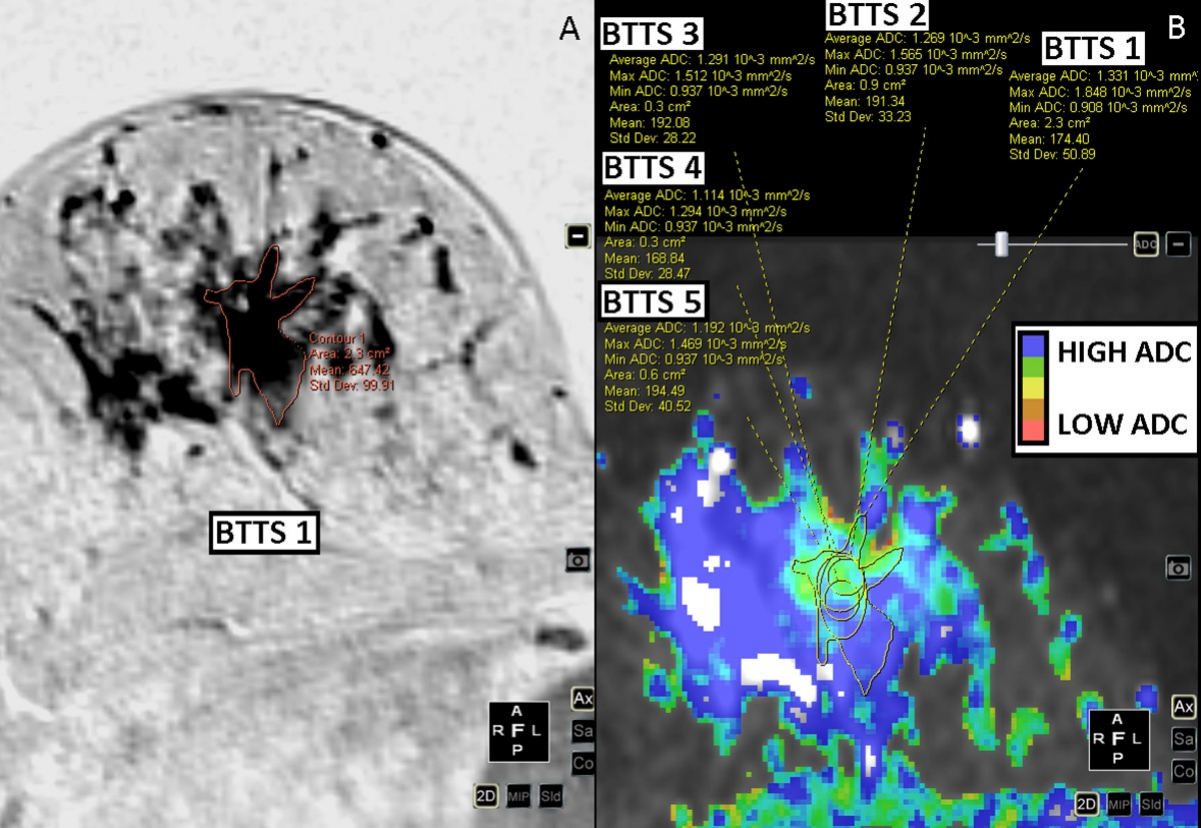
Sclerosing adenosis, columnar cell changes and apocrine hyperplasia of the right breast. MRI guided biopsy proven and unchanged in 4 years of follow-up. DCE-T1 subtracted images (inverted) (A) and DWI b0-1000 images (B). BTTS = Breast tumor tissue selection.
